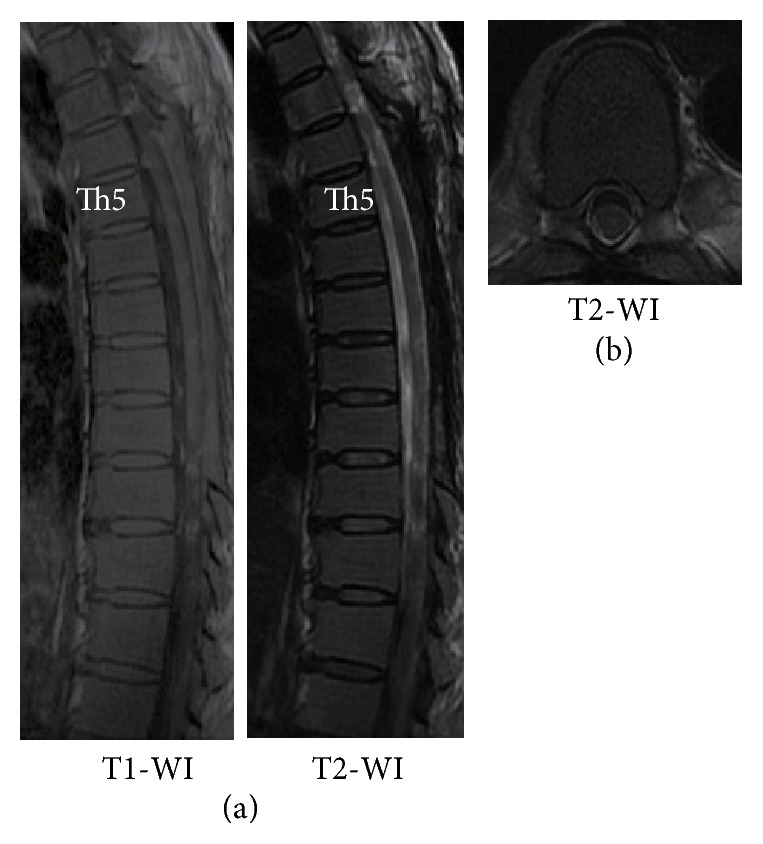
MRI taken one year later shows no recurrence. (a) Sagittal plane. (b) Axial plane.
